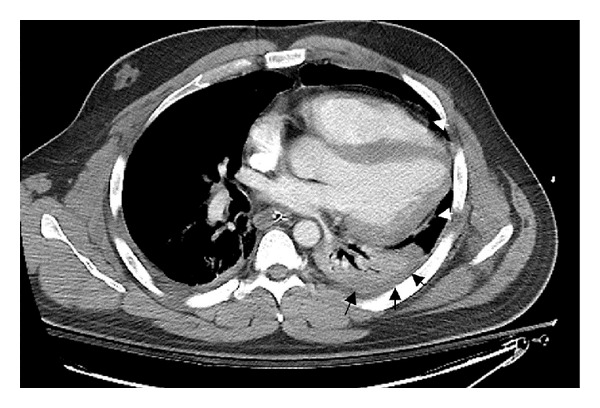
The CT study performed upon emergency department arrival. The heart and mediastinum are dramatically displaced to the left. The heart has migrated posteriorly to rest on the atelectatic left lower lobe of the lung (black arrows). The white arrows demarcate the edges of the pericardium through which the heart has herniated.
